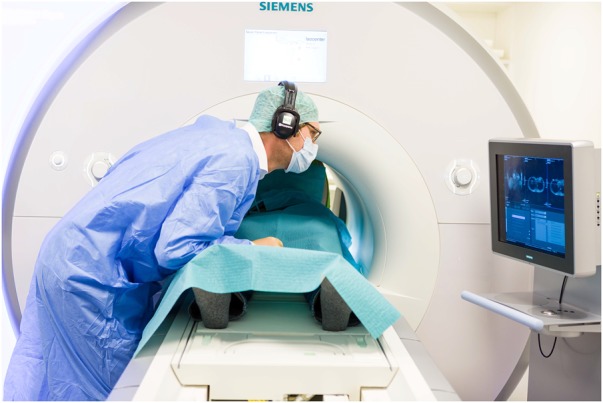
Current situation: A radiologist performs an MRI-guided needle puncture while using a separate display outside the MRI bore
